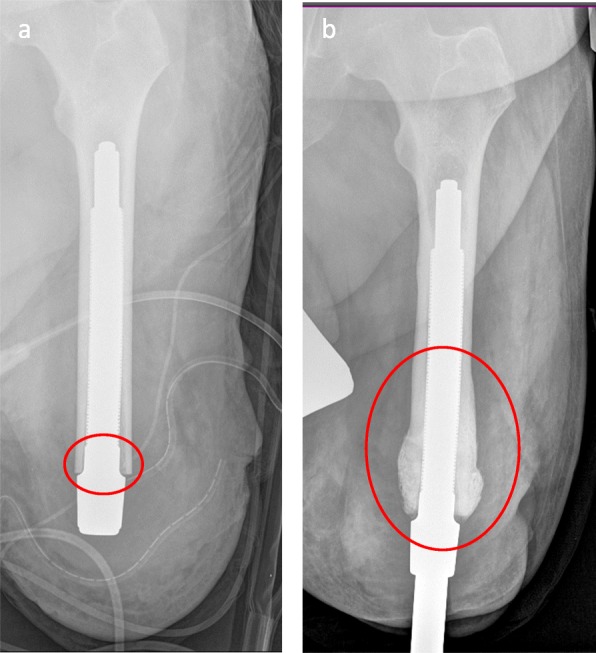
a, b Postoperative x-ray after Endo-Exo-Prosthesis. The area marked with a red circle (small) depicts the bone-implant transition area that is not covered with tripods. b Example of osseous hypertrophy at distal end of femur in the 3-year follow-up including the implant transition area that is not covered with tripods (big red circle)
